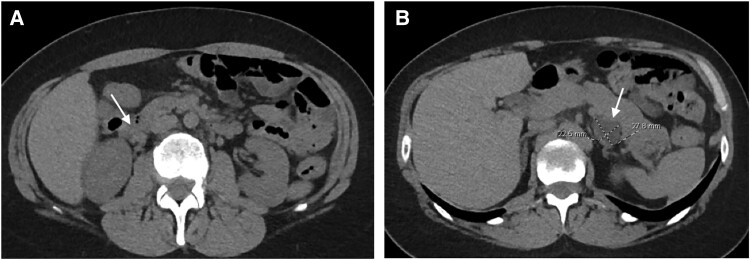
(A) Patient B's CT abdomen/pelvis noncontrast showing hyperplasia of the right adrenal gland. (B) Patient B's CT abdomen/pelvis noncontrast showing a left adrenal nodule. Abbreviation: CT, computed tomography.
